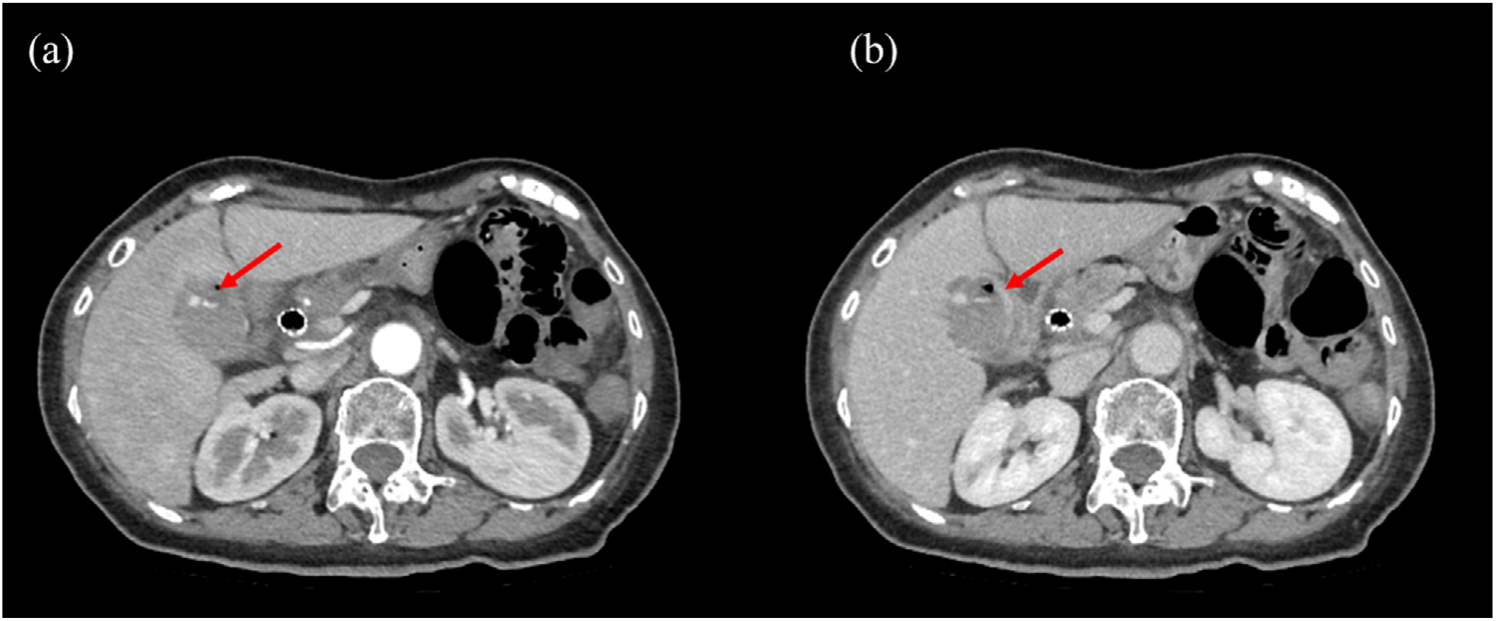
(a) Contrast‐enhanced computed tomography (arterial phase) showing rupture of a cystic artery pseudoaneurysm (arrow). (b) Contrast‐enhanced computed tomography (equilibrium phase) showing rupture of a cystic artery pseudoaneurysm (arrow).
